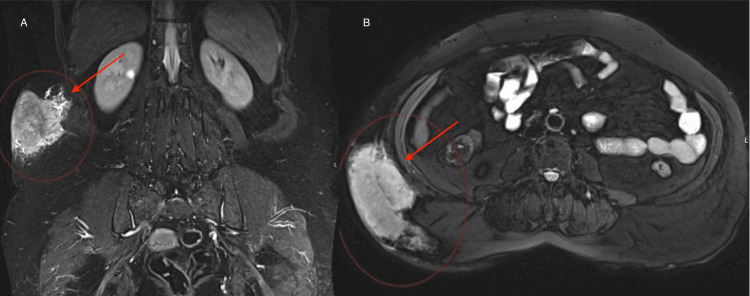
MRI of the abdomen and pelvis showing sagittal (A) and cross-sectional (B) multilobulated subcutaneous soft tissue lesion at the right subcutaneous posterolateral abdominal wall adjacent to the lumbar spine measuring 10 × 5 x 8 cm
